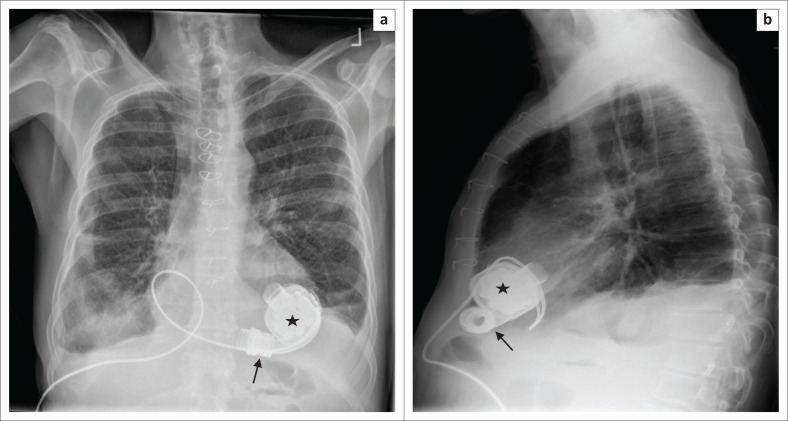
Frontal (a) and lateral (b) chest radiographs showing a HeartMate III device implanted in a 58-year-old male with severe left ventricular dysfunction. Note that the HMIII device has an angled outflow cannula (arrow) and a circular impeller unit (star).
